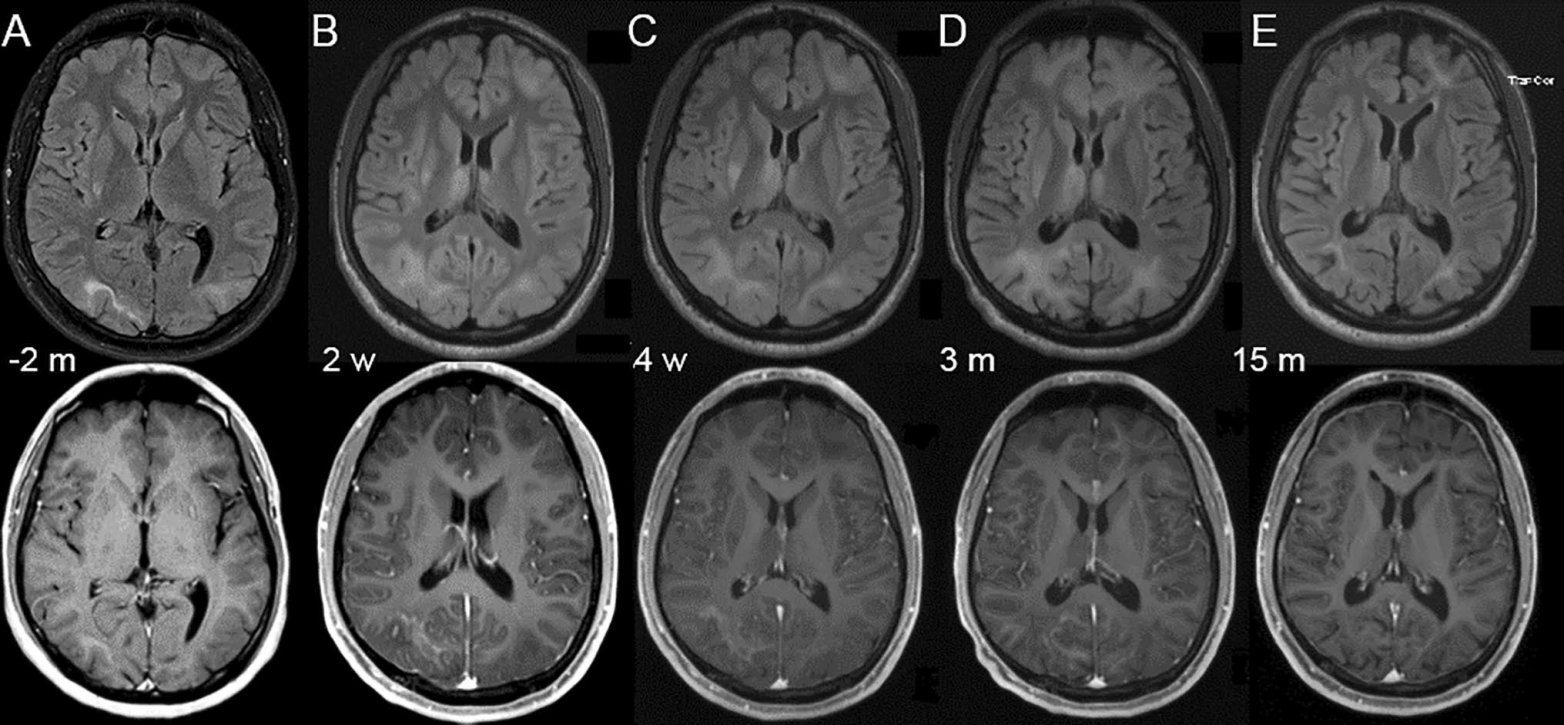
Transiently contrast-enhancing lesion in Patient 1. A Two months before treatment, bi-occipital lesions with minimal contrast enhancemend on the right as well as a lesion in the globus pallidus are visible. B Lesions progress after treatment, a new lesion in the left frontal lobe, as well as bi-thalamic lesions are visible. Contrast enhancement is increased in the right occipital lesion. C Lesion in the thalamus is less intense, otherwise stable MRI. D + E Declining intensities of lesions, no contrast enhancement is detectable 15 months after initial treatment. Top row: *FLAIR* (Fluid-attenuated inversion recovery) MRI sequences, bottom row: *MP RAGE* (magnetization-prepared 180 degrees radio-frequency pulses and rapid gradient-echo sequences) MRI sequences post gadolinium application. *m* month,* w* week
